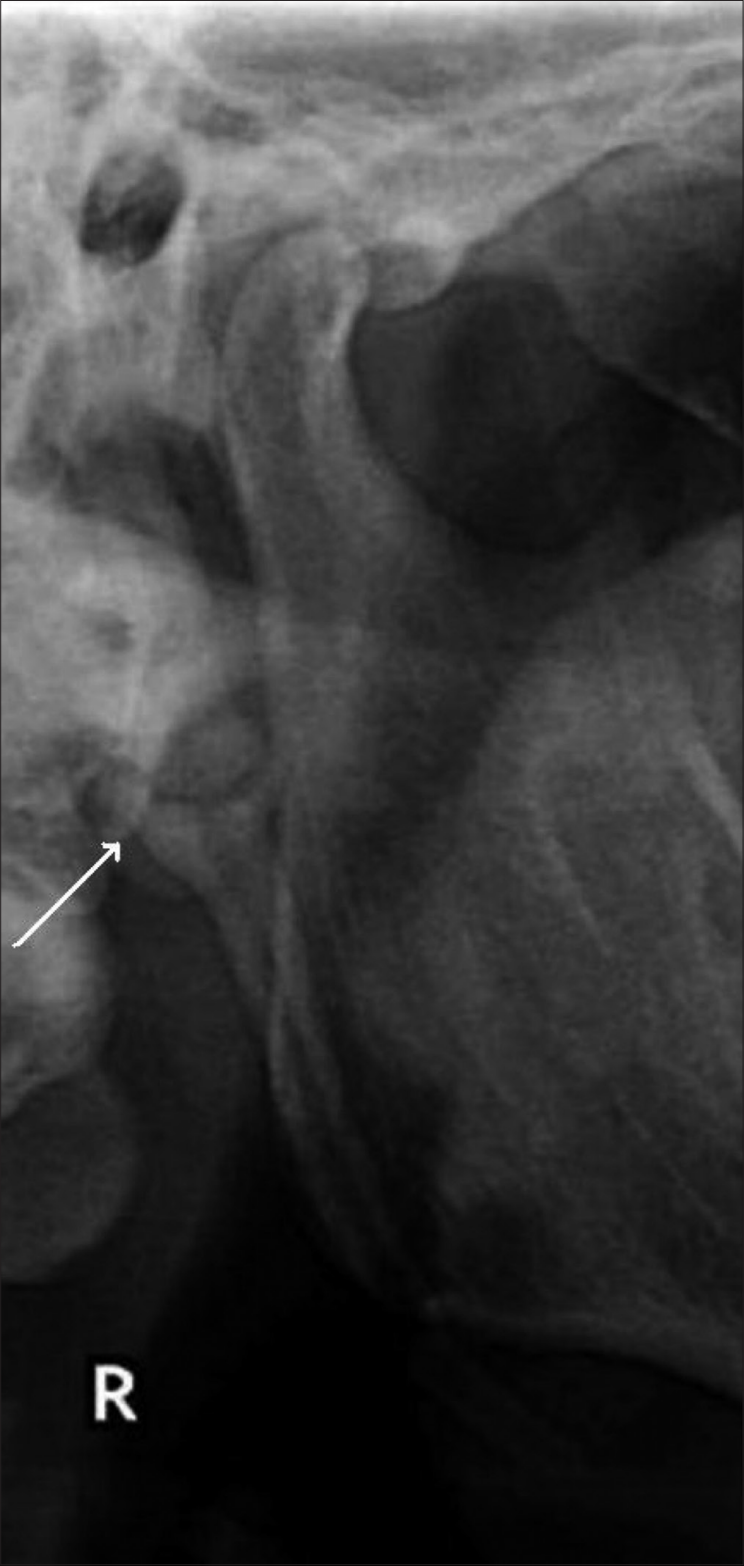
The orthopantomogram shows a Langlais type II styloid process (arrow)
